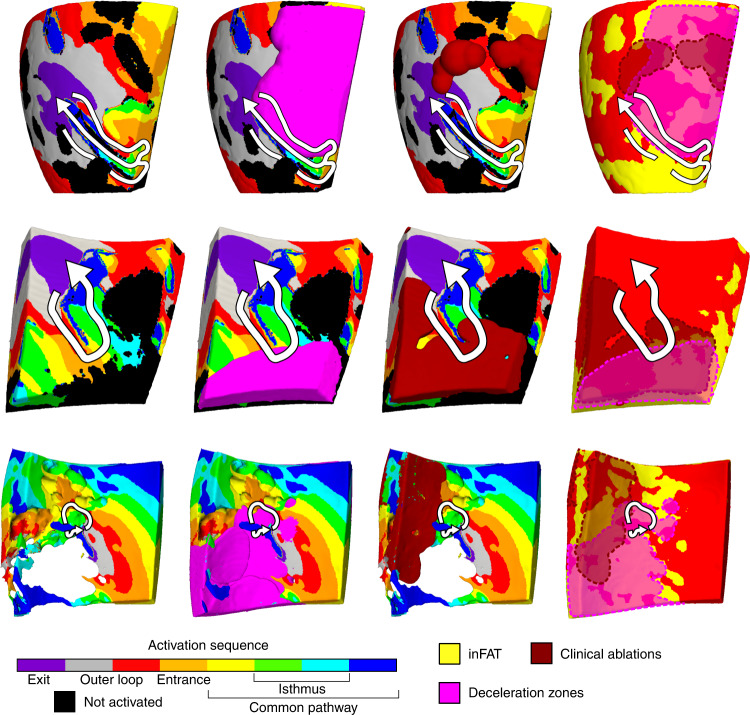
inFAT promotes conduction abnormalities in critical sites of the VT circuitry. Three examples of VT circuits in hybrid CT-MRI heart models with the overlapping clinical ablations and DZs are displayed. For these three VT circuits, there was inFAT but little to no fibrosis present. Arrows trace the re-entrant pathway from the exit site to the end of the common pathway. The pink regions denote the DZs, and the dark red denotes the estimated volume of the ablation lesion. Non-activated tissues were omitted for the bottom VT circuit to better visualize the intramural activation sequence.
